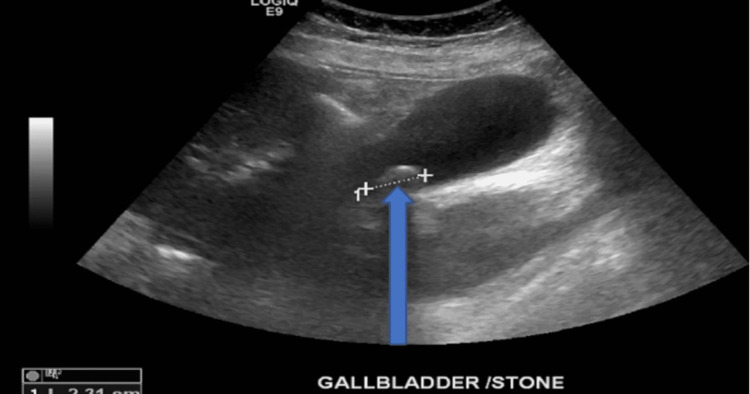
Abdominal ultrasound taken during patient admission Abdominal ultrasound is showing the gallbladder with a single solitary stone measuring 2.31 cm as indicated by the blue arrow cm, centimeter
